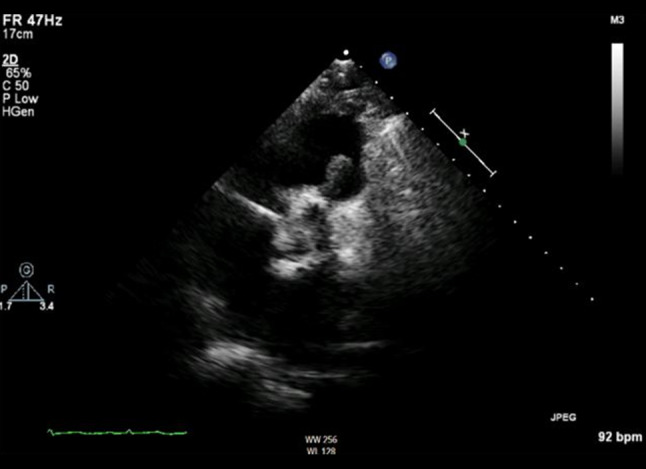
TTE showing a mobile structure on the pulmonary valve. *TT* transthoracic echocardiography
